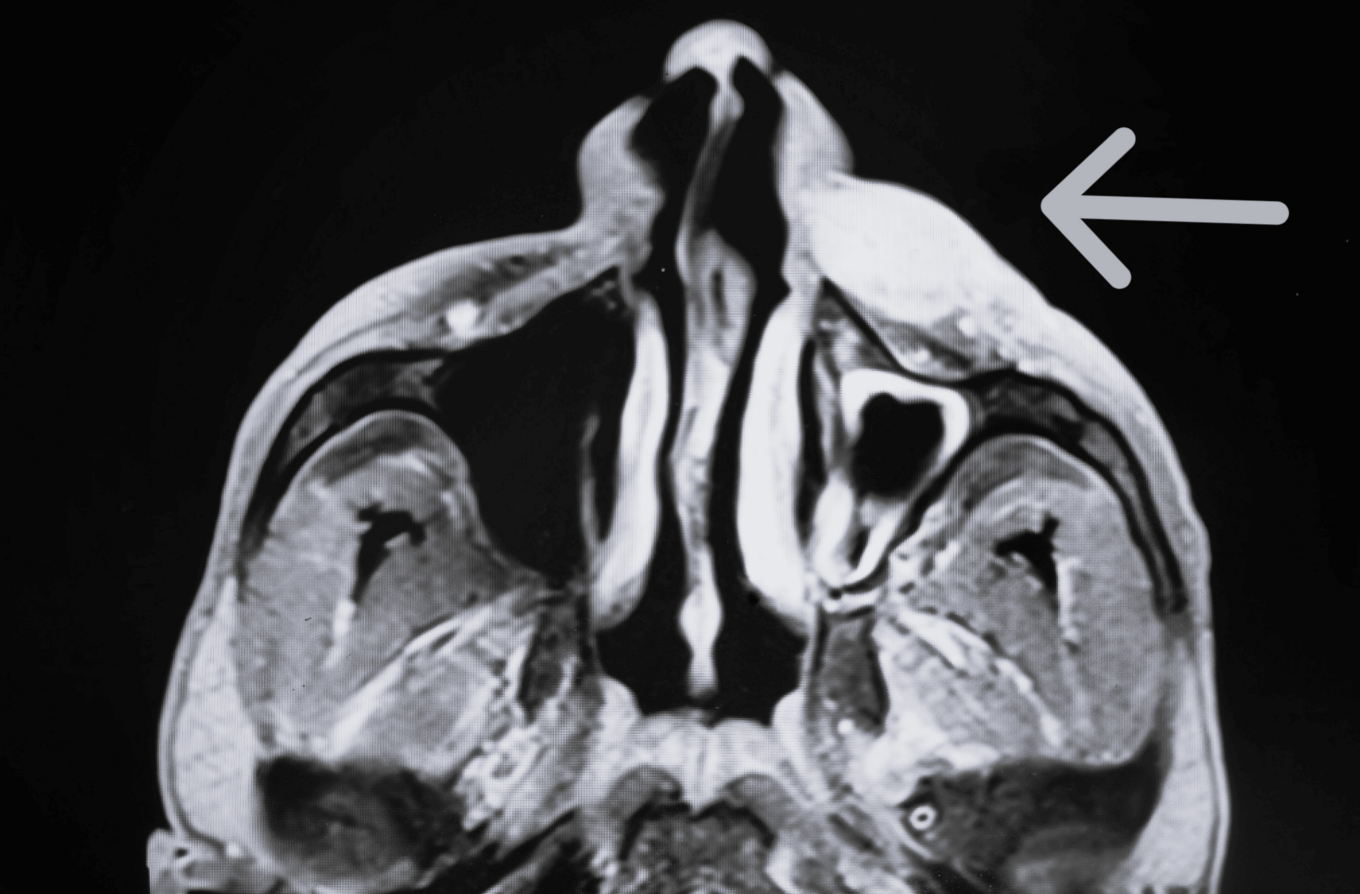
Axial view of MRI of the lesion indicated by the white arrow
